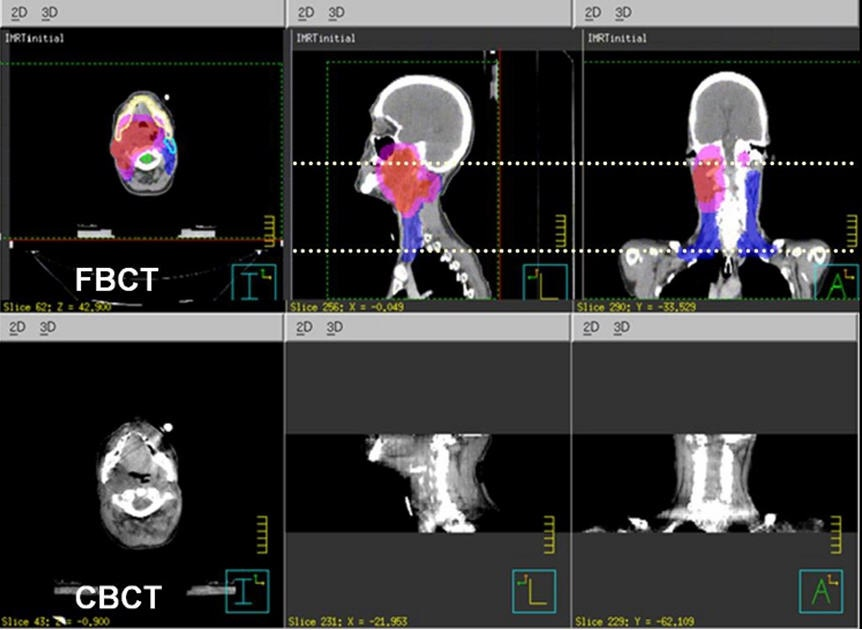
An example where the targets, shown in watershed on the planning FBCT (a), go beyond the maximum longitudinal coverage of the CBCT (b).
